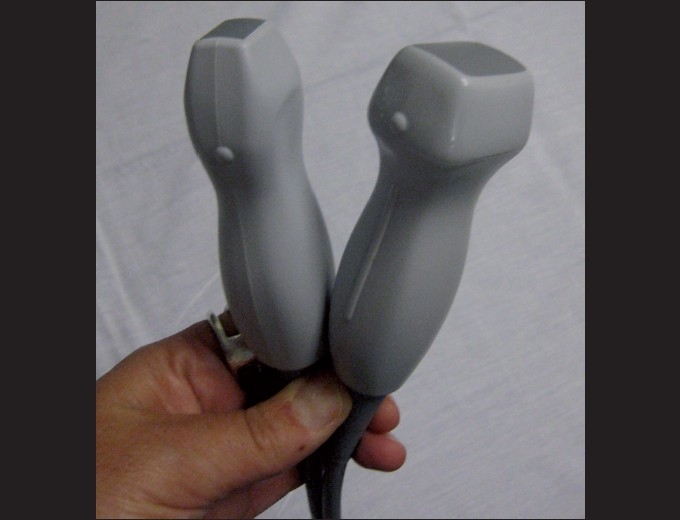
Curvilinear transducers (2-5 MHz) ideal for bedside cardiac utrasonography. Note the small footprint that can easily scan in between the rib spaces
